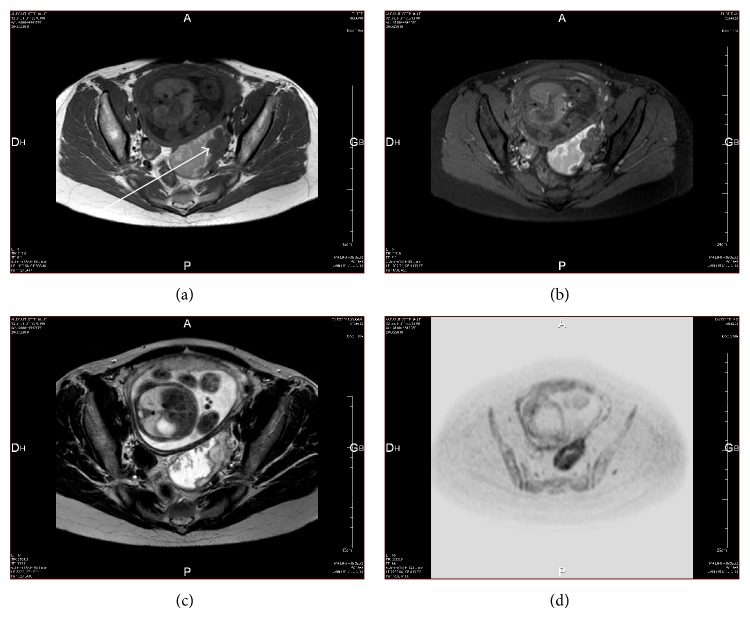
Magnetic Resonance Imaging features of the left decidualized ovarian mass. Axial T1 weighted image (a) demonstrates an intermediate signal intensity of the solid component in the left ovary (arrow), which remains unchanged on fat suppressed T1 weighted image (b). The solid component exhibits an intermediate T2 weighted signal (c) and a high diffusion-weighted signal (d).
